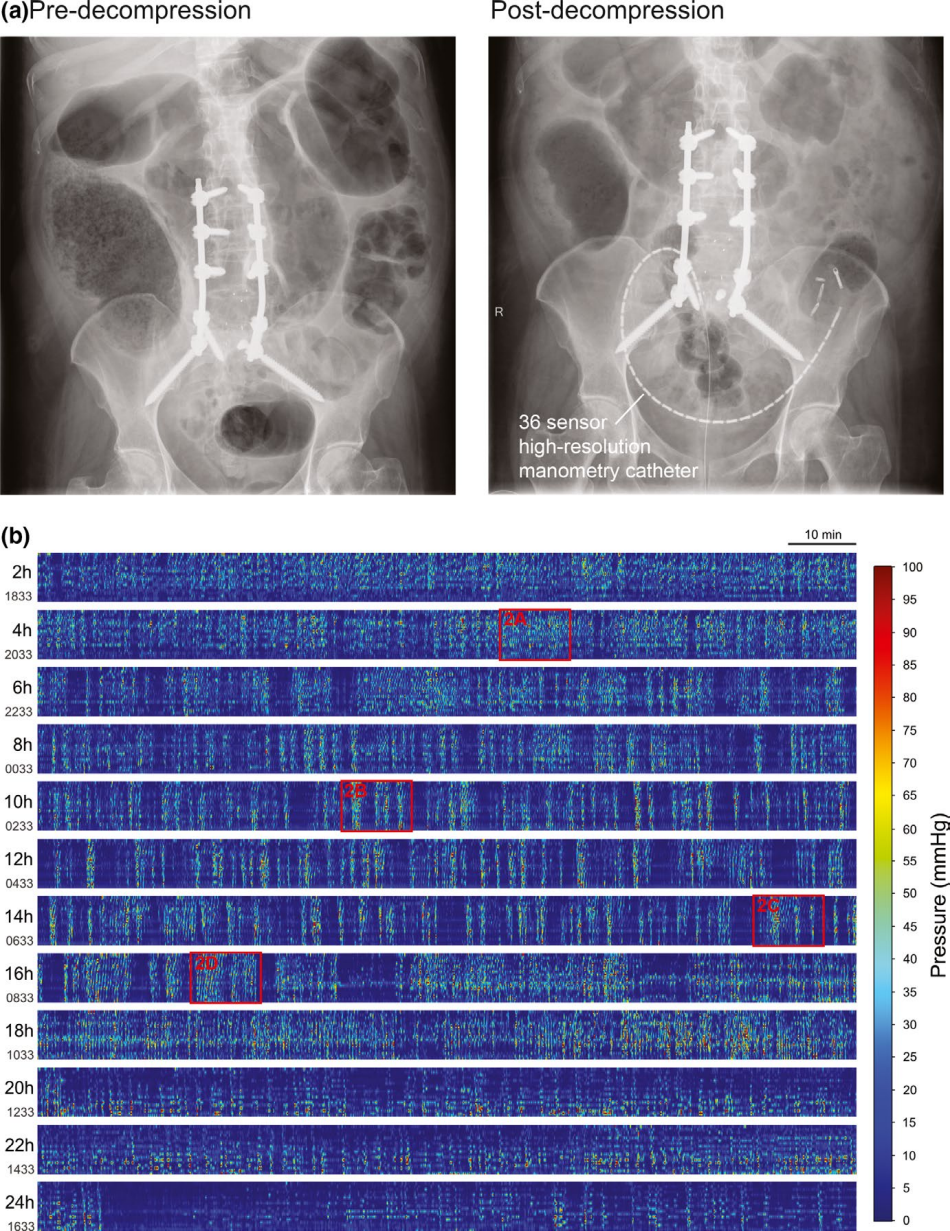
(a) Abdominal radiographs prior to and following endoscopic decompression and insertion of high‐resolution manometry catheter. (b) Overview of the entire 24 h recording period showing marked distal colonic hyperactivity. Each horizontal bar represents a 2 h period. The time of day at the start of each 2 h block is shown on the left of the figure. Red boxes represent periods of data displayed in Figure 2
